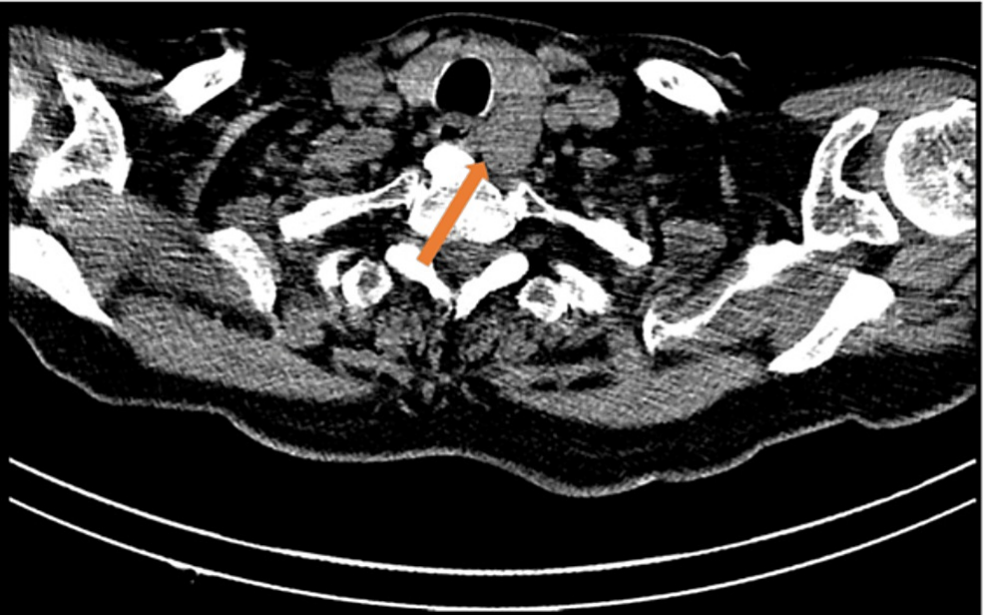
Nuclear medicine parathyroid SPECT – axial image Oval-shaped soft tissue mass posterior to the left thyroid lobe (orange arrow). SPECT - single photon emission computed tomography
